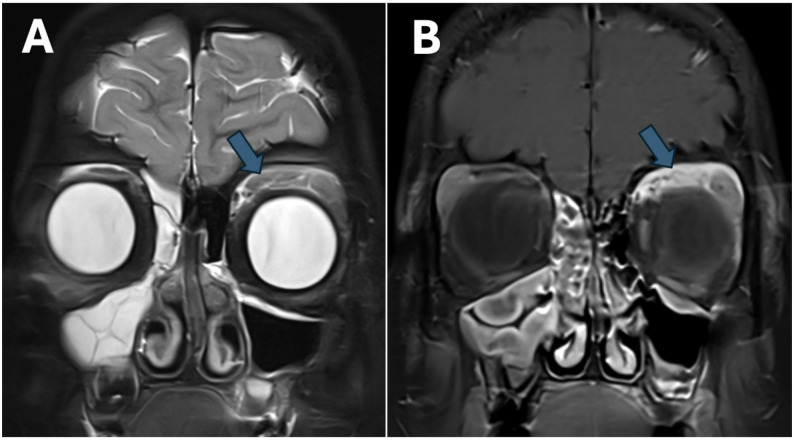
MRI: coronal T2-weighted turbo-inversion recovery magnitude (TIRM) image (A) and coronal T1-weighted image with fat saturation following gadolinium administration (B) demonstrate a T2-hyperintense mass with strong post-contrast enhancement (arrow)
